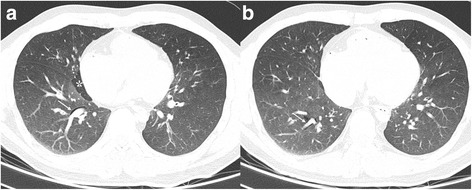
Post mortem computed tomography. High-resolution lung images show distended lungs with extensive bilateral air trapping, sometimes heterogeneous as pointed in *, associated with a decrease in vascularity. PIE dissects peribronchovascular interstitium in the right lower lobe, besides segmentary (a) and subsegmentary (b) bronchi. There is also bronchial wall thickening with associated narrowing of bronchial lumen.
